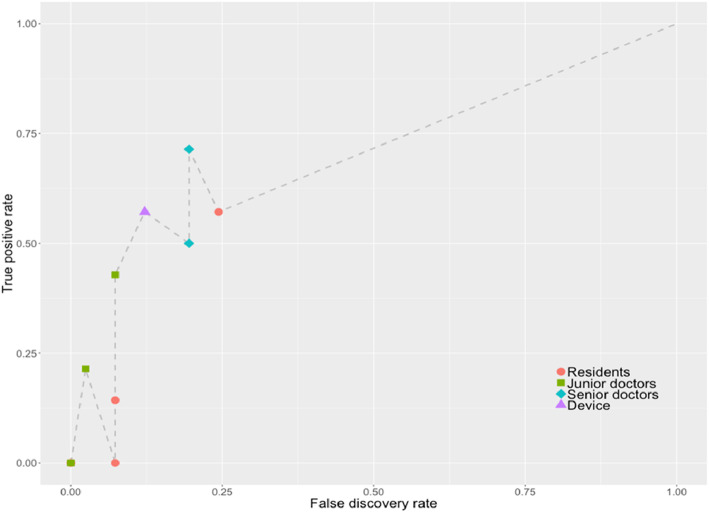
Receiver-operating characteristic (ROC) curves for e-ASPECTS shows performance of the device and every doctor included in this study
